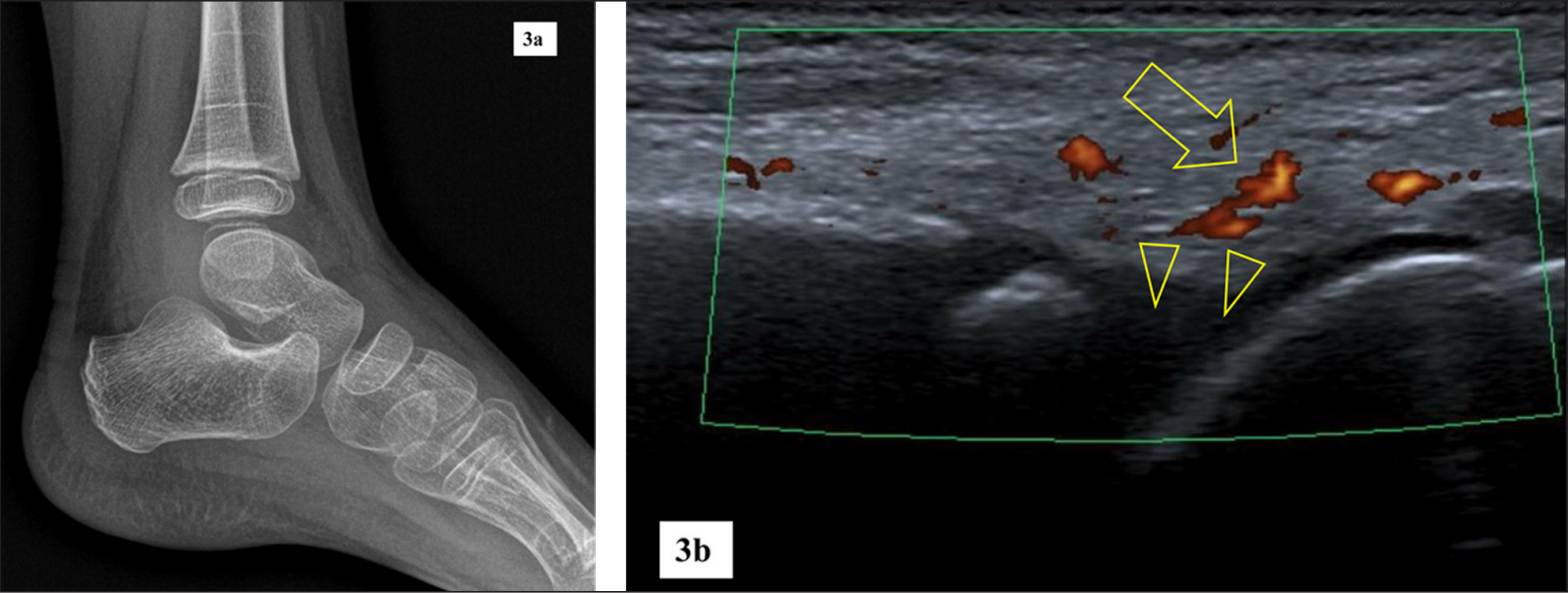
5-year-old girl with confirmed JIA. a. Lateral radiograph of a left ankle is unremarkable. b. Sagittal ultrasound of the left ankle shows hypervascular synovium on Power Doppler mode (arrow) and small tibiotalar effusion (arrowheads).
